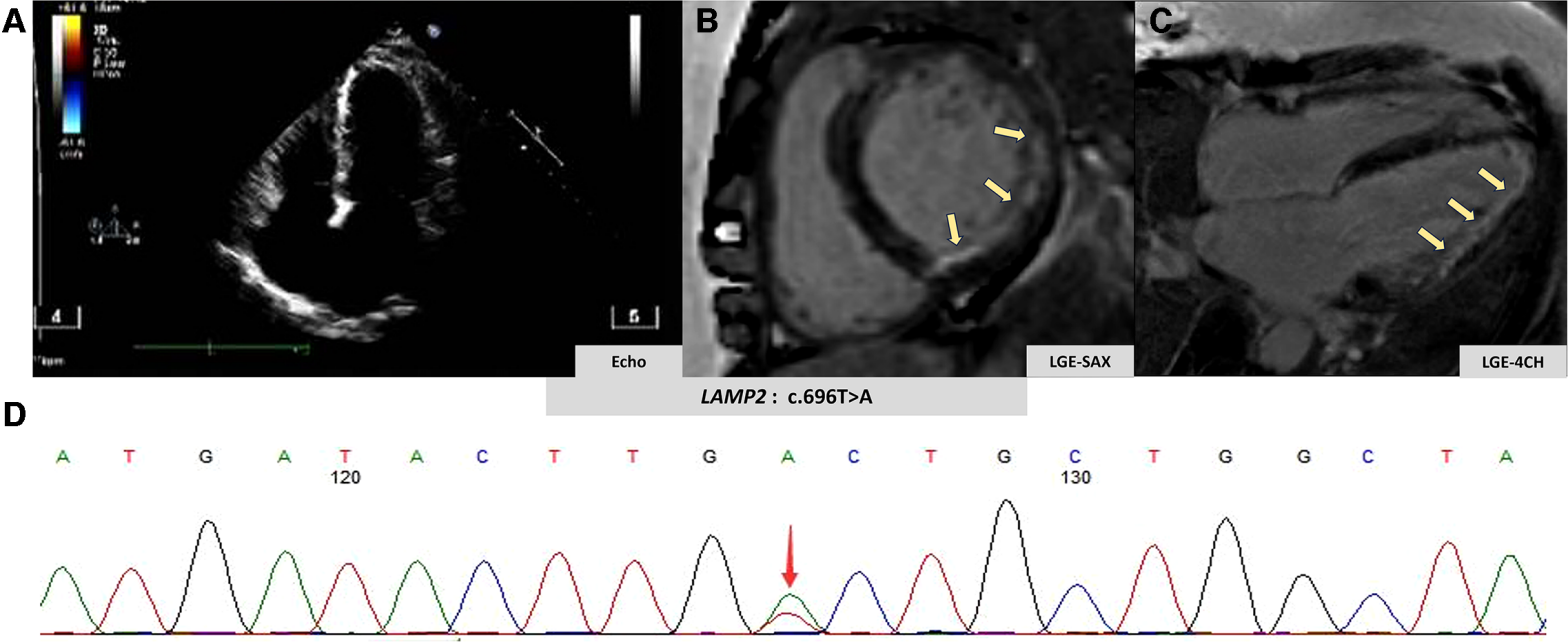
Imaging and genetic findings in Danon disease. (A) Patient's echocardiography view. (B,C) Patient's late gadolinium enhancement by CMR (yellow arrow). (D) Genetic analysis of the clinically affected member.
